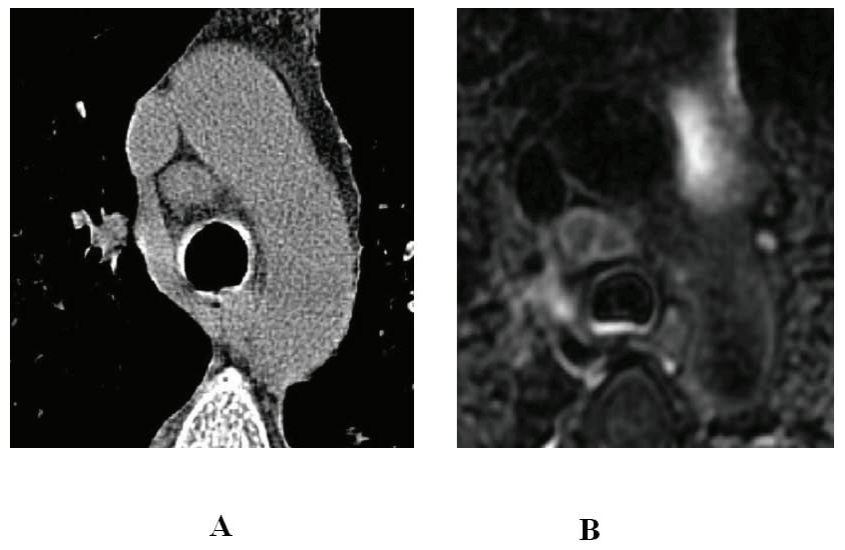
53 year-old woman with pulmonary tuberculosis. A) HRCT, reconstructed for mediastinum, shows an enlarged pretracheal lymph node, B) Unenhanced MRI also depicts lymph node caseosis.
